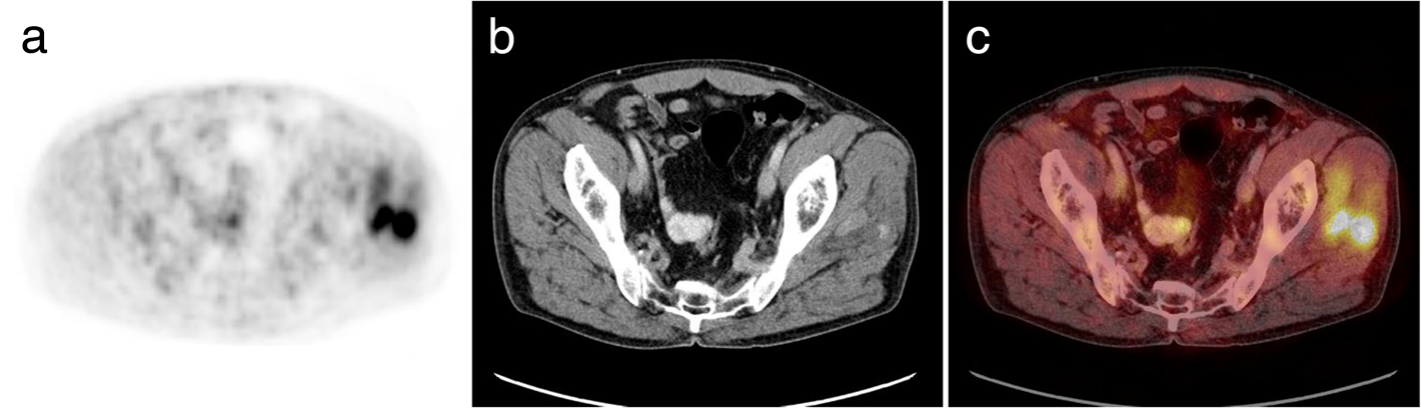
Imaging findings in a patient with lung cancer. PET (a) showing focal uptake in the left gluteal musculature (arrow). CT scan (b) detecting intramuscular masses in this area (arrow). Fusion image (c).
